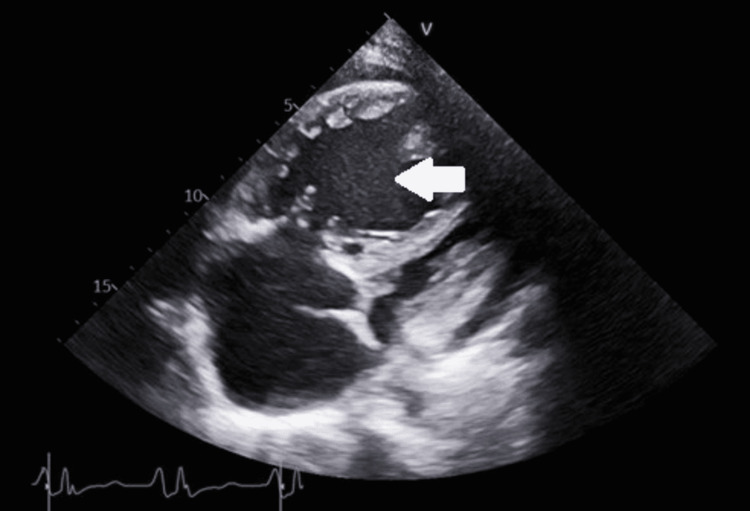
Case 1 transthoracic echocardiogram subcostal view. The arrow indicates dilated right ventricle comparable in size to the left ventricle.
